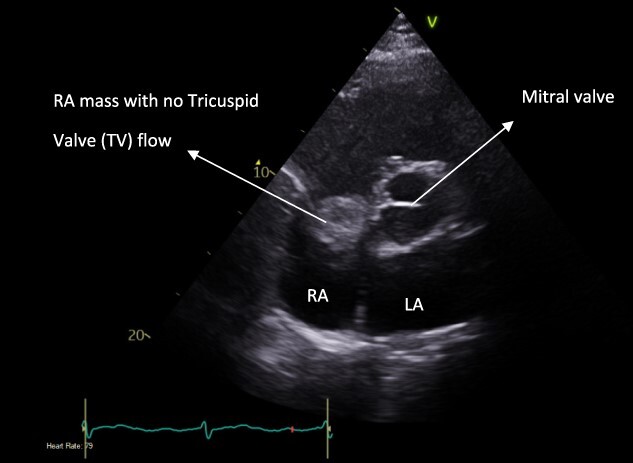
Pre-operative apical four-chamber view echocardiogram showing RA bulk blocking TV flow.
